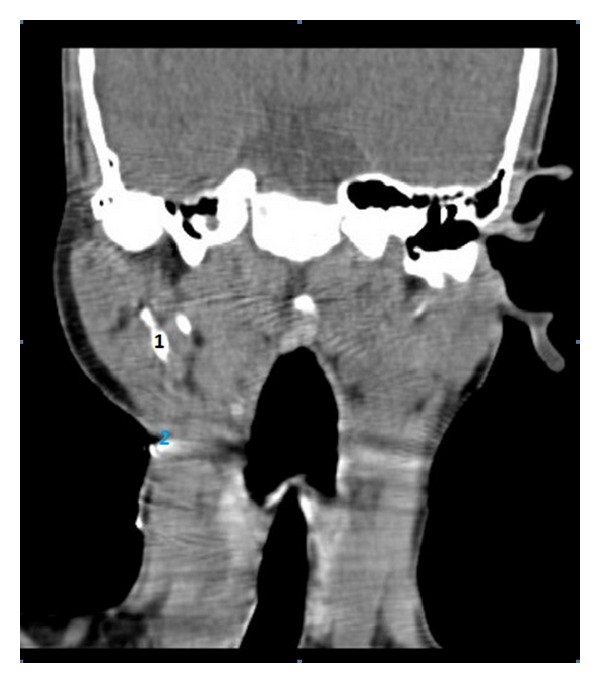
CT fistulogram with coronal reconstruction showing the fistula tract (marked as 1) passing through the deeper part the parotid gland. The cutaneous opening is marked as 2.
